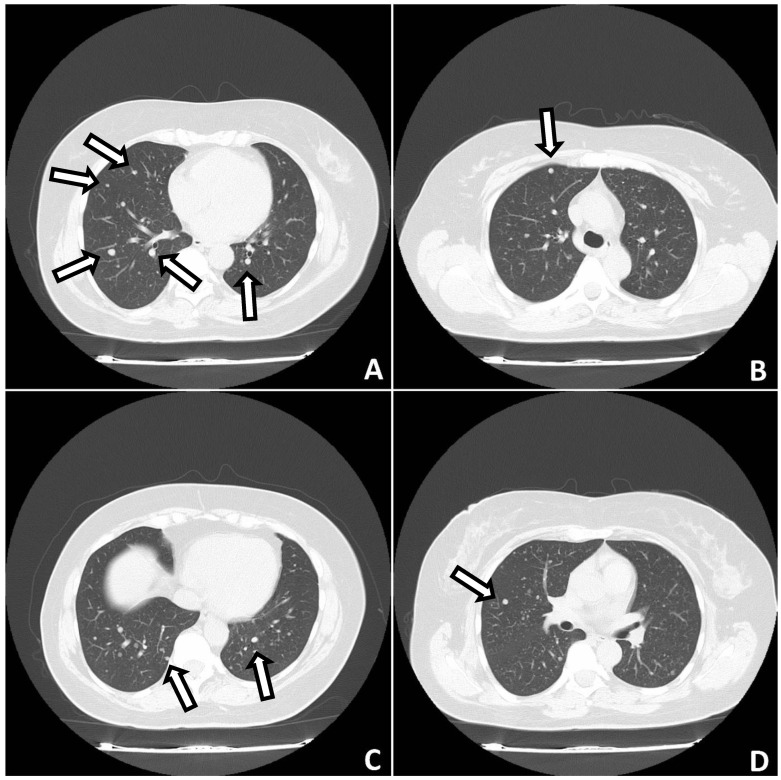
CT of the lungs. Lung nodules are visible in both lungs (arrows) (A–D).
